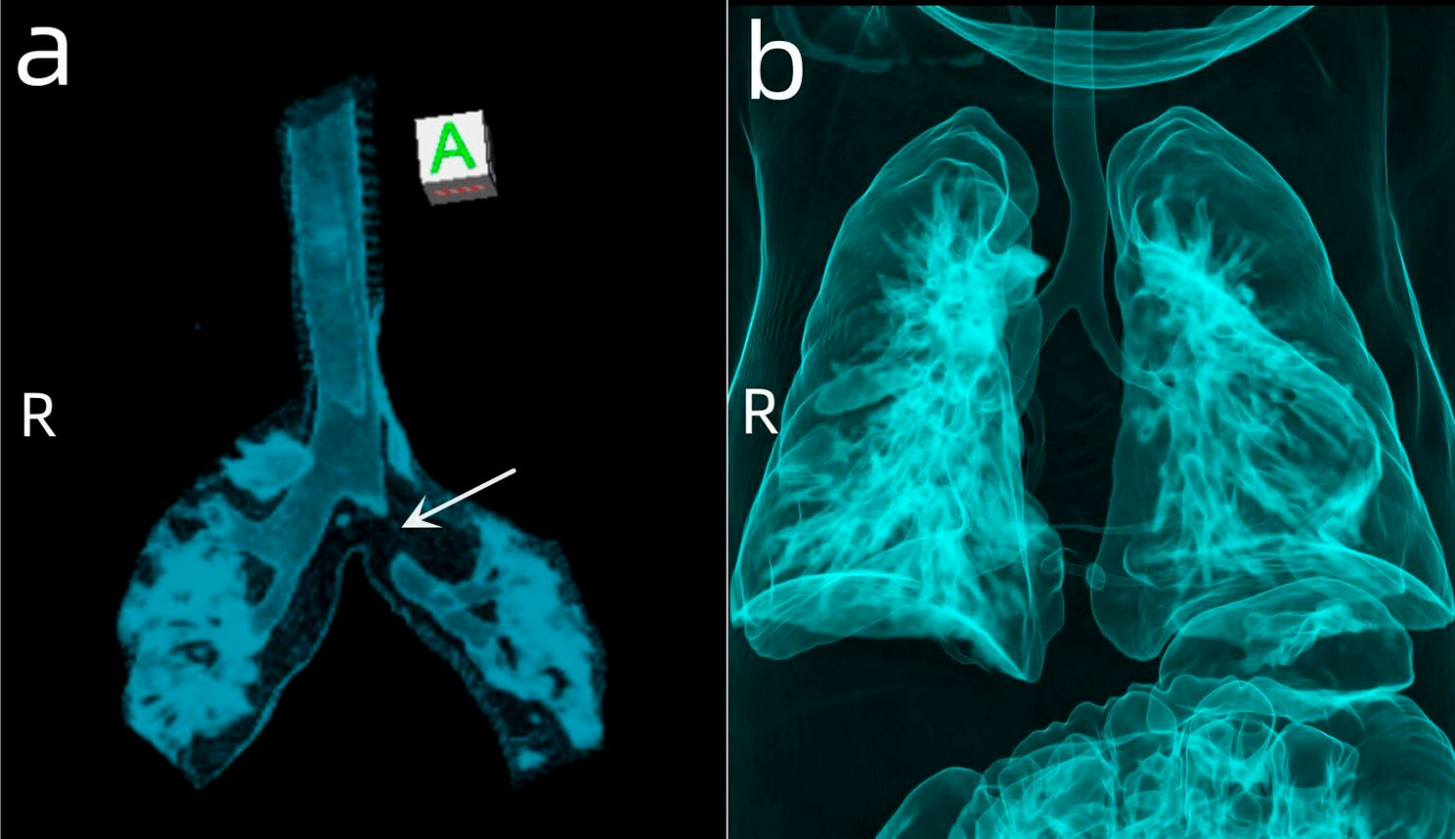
Three-dimensional reconstruction of the CT scan shows that the airway stenosis was relieved after surgery. Before surgery (A), after surgery (B)
